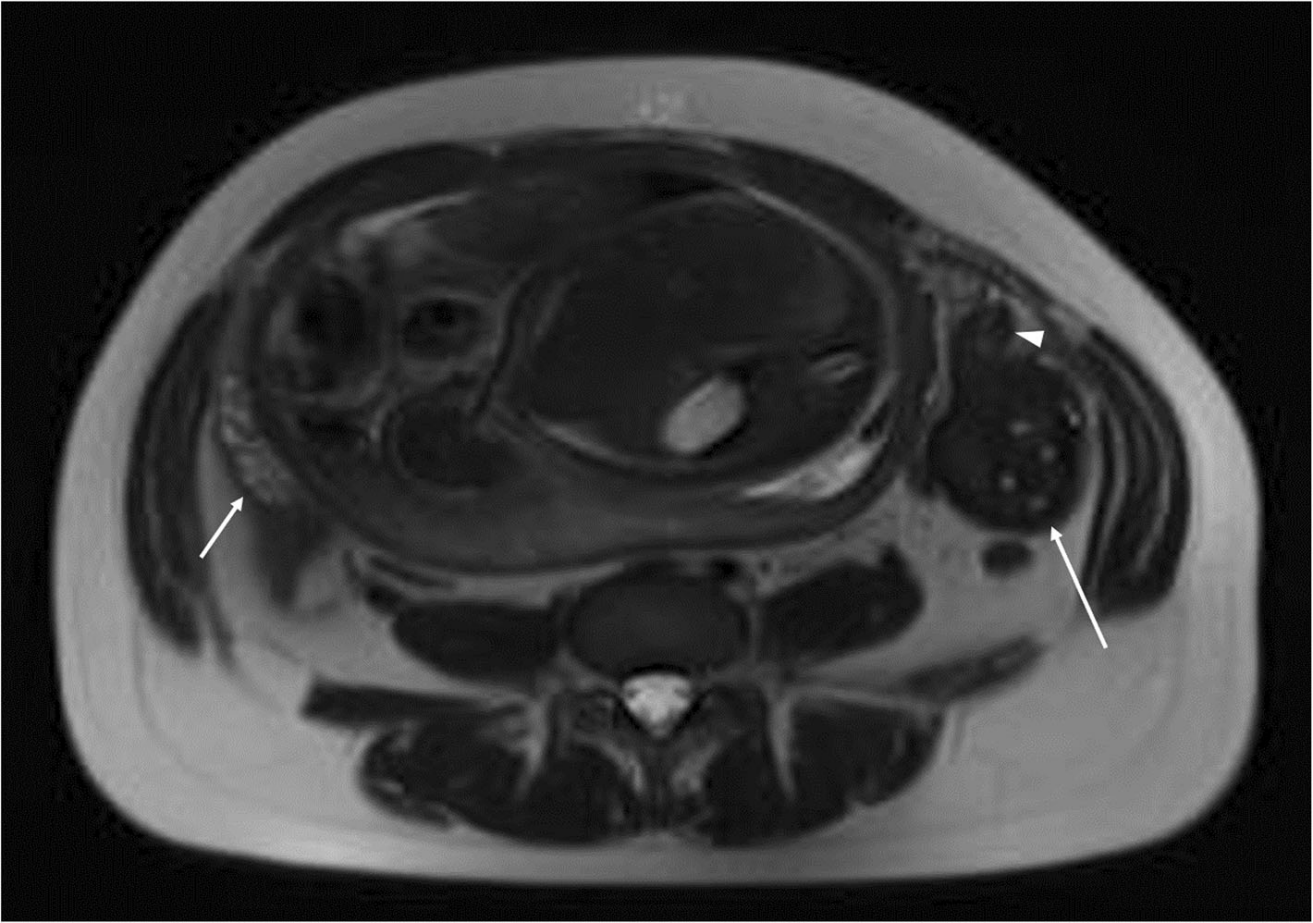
Axial T2-weighted magnetic resonance imaging (MRI) shows an enlarged left ovary (long arrow) with multiple follicles and extensively low signal intensity compared with the normal right ovary (short arrow). The adjacent left fallopian tube was found to be thickened (arrowhead) with the diameter of 11 mm
